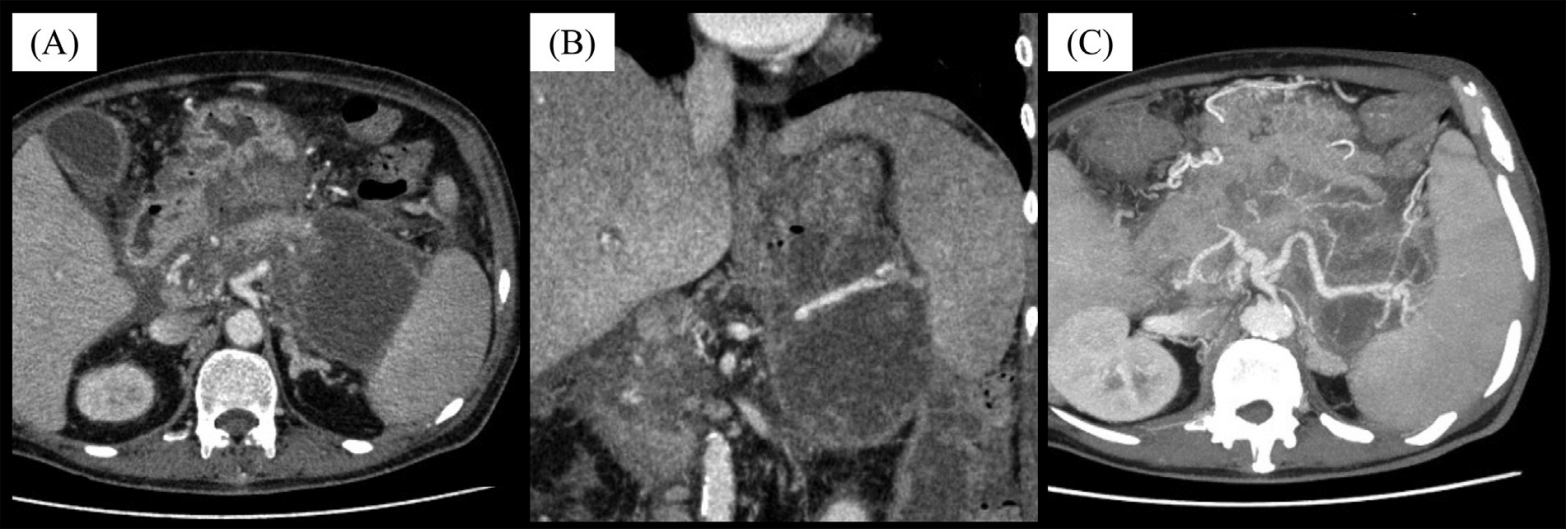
Contrast-enhanced CT at the time of transfer to our hospital. The walled-off necrosis (WON) is demonstrated around the pancreas in the (A)axial and (B) coronal views. The splenic artery is entirely distributed inside the WON in the (C) maximum intensity projection image.
